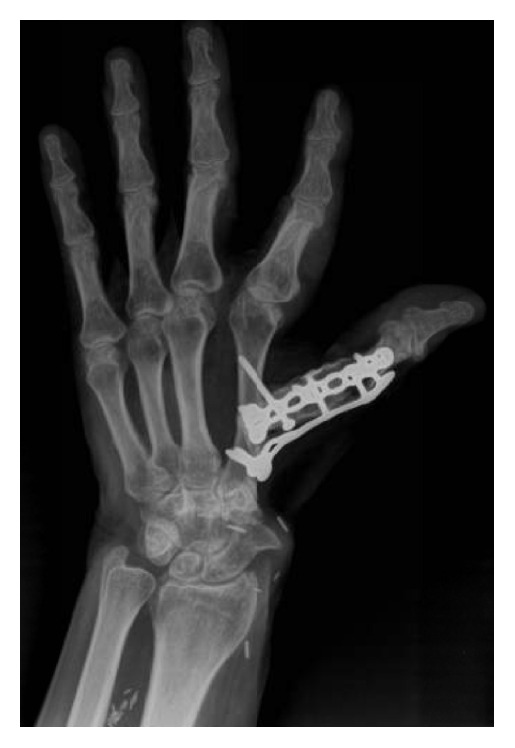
Postoperative X-ray of the left hand demonstrating fusion of the proximal phalanx of the thumb to the second metacarpal.
